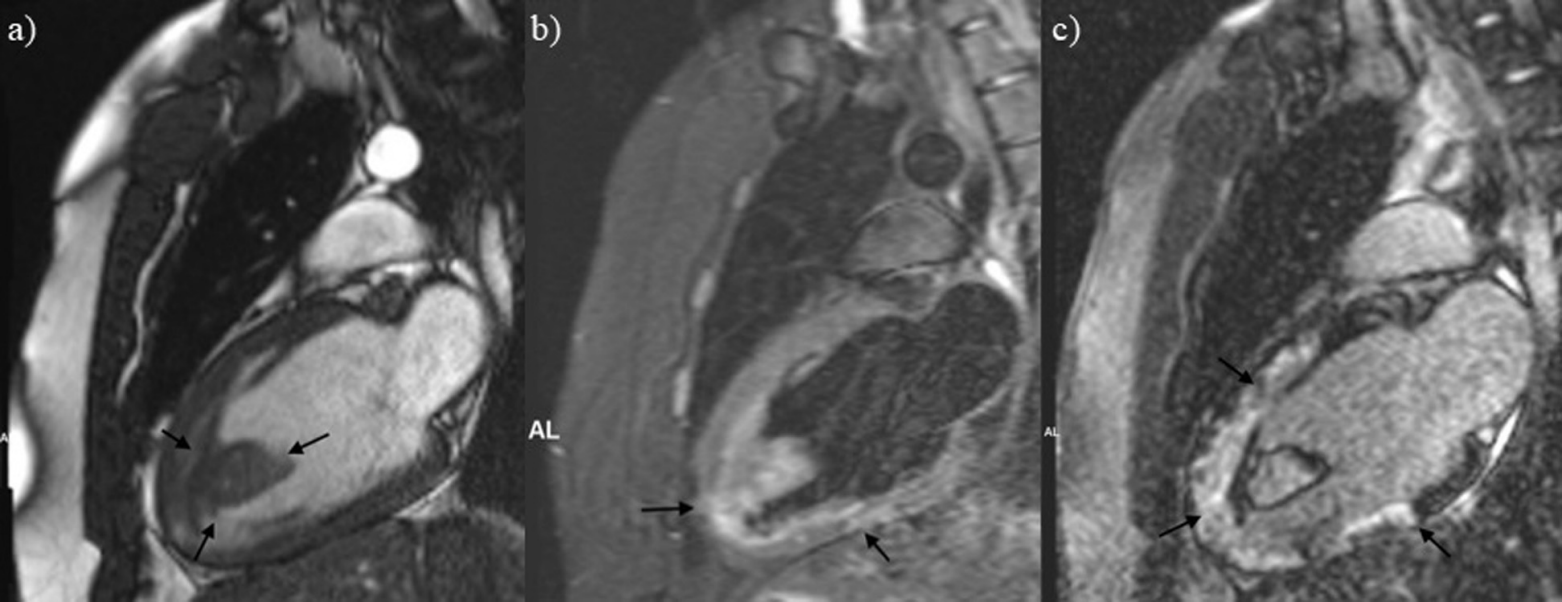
Cardiovascular magnetic resonance imaging two-chamber view. (A) Large wall-adherent apical thrombus, which appears isointense to myocardium (*arrows*) on a CINE (TruFISP) image still. (B) Apical thrombus, which appears hyperintense to myocardium and with areas of mild patchy edema within the LV apex and inferior wall (*arrows*) on a triple inversion recovery image. (C) Large areas of transmural and midmyocardial delayed myocardial enhancement reflecting areas of necrosis/fibrosis (*arrows*) on a delayed postcontrast image.
